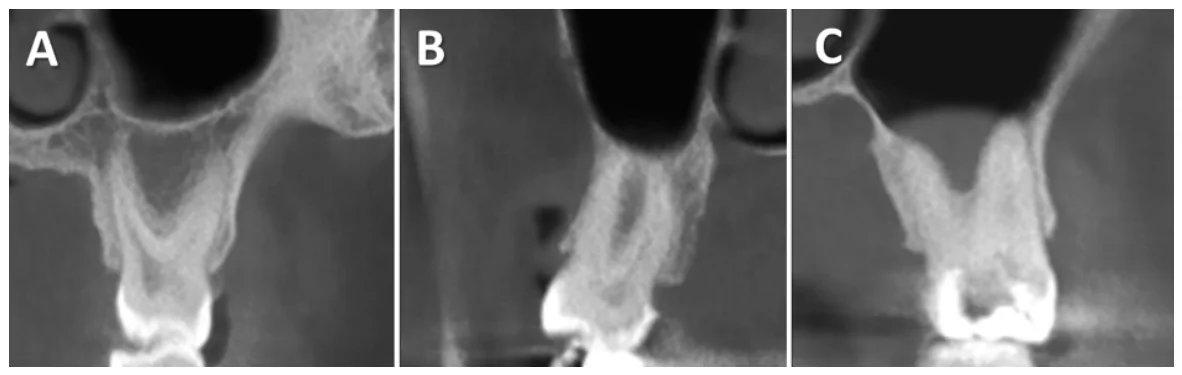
The relationship between the maxillary sinus and roots on coronal CBCT scans. (A–C) Same as Figure 1.
